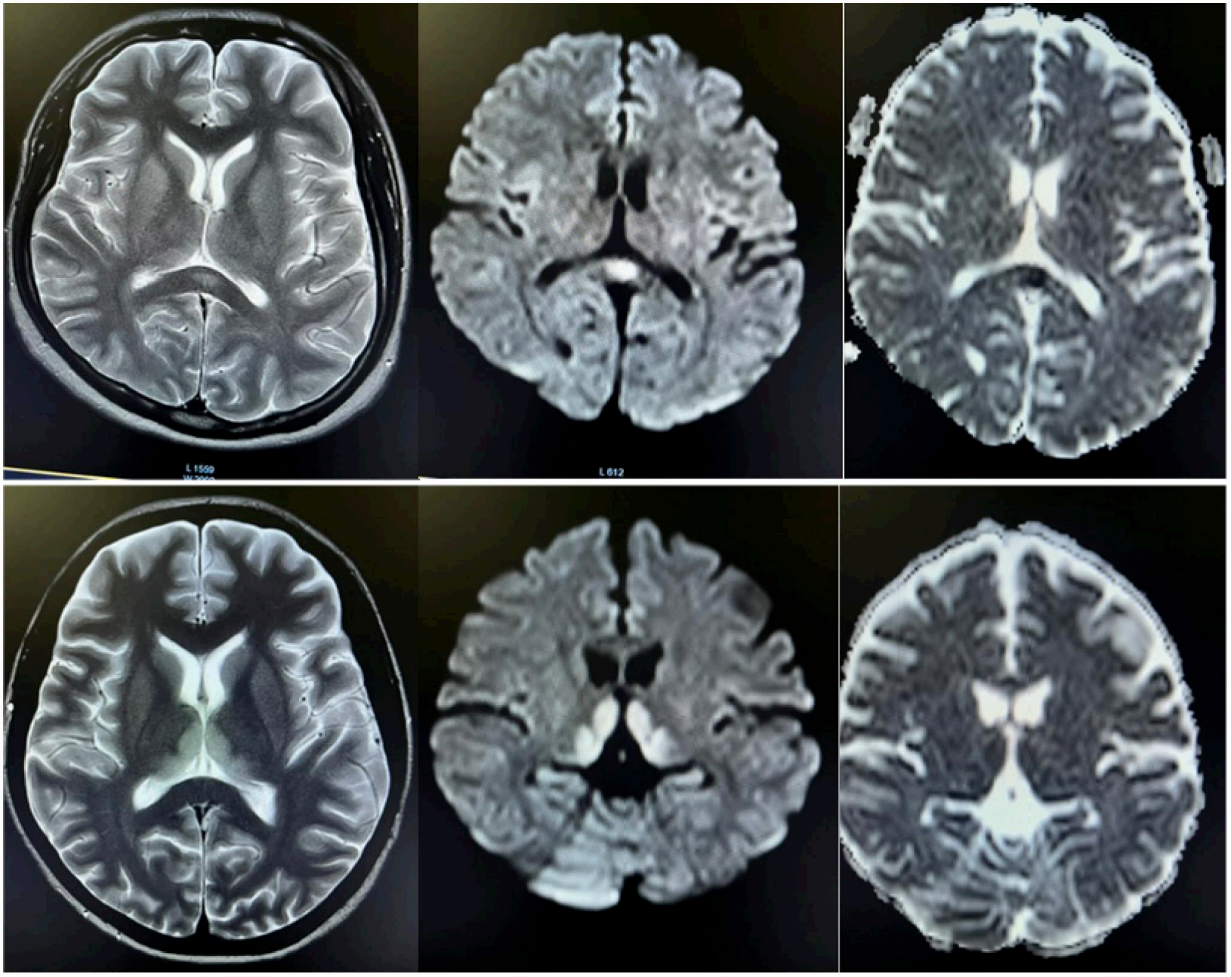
Small well-defined ovoid lesion in the midline within the splenium of the corpus callosum and bilateral thalami and high signal on T2 and FLAIR with no enhancement on postcontrast sequence. The DWI/ADC sequence shows mild restricted diffusion.
